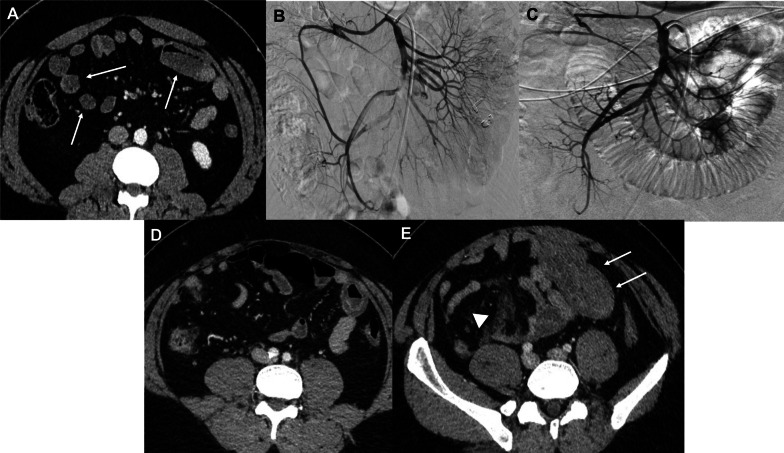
A 25-year-old male patient presented with early acute mesenteric ischemia (embolus of the superior mesenteric artery, SMA). Decrease in enhancement of the small bowel (arrow) compared to non-ischemic ones (arrowheads) on baseline CT portal-venous phase (a) with complete occlusion of the distal branches of the SMA (b). Complete recanalization of the SMA by thrombus-aspiration and in situ thrombolysis (c). Contrast-enhanced CT (portal venous phase) performed 12 h after revascularization for systematic post-procedural evaluation showed a thickened jejunal loop (arrow) with hyperenhancement of the mucosa and edema of the submucosa consistent with reperfusion injury (d). 24 h after revascularization, the patient has bloody diarrhea with anemia. On portal-venous phase CT, the previously thickened small bowel segment showed marked thickening with intra-luminal hyperattenuating material consistent with hemorrhage (arrow) and mesenteric fat stranding (arrowhead) (e). The patient was closely monitored surveillance, and no laparotomy was needed
